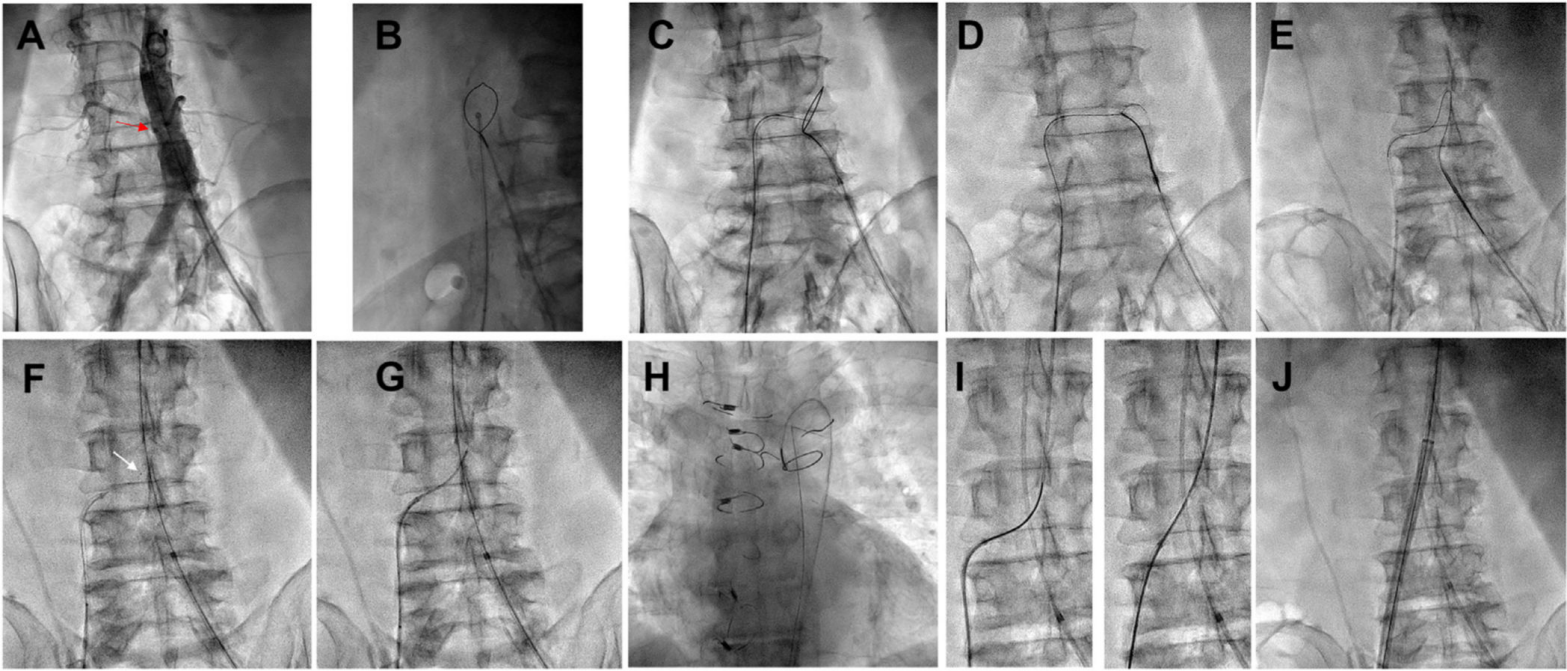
Traversal Sequence Representative traversal sequence. (A) Aortogram in computed tomography–derived projection. No venogram is required. Arrow indicates target. (B) Orthogonal projection confirms that the traversal system aims at the snare. (C) Electrified guidewire exits the inferior vena cava and enters the aorta. Electrification ceases when the wire crosses the snare border. (D) The aortic guidewire is ensnared while feeding forward to avoid outward traction on the aortic catheter. (E) The aortic guide is advanced, leading with a looped guidewire. (F) A 0.014-inch microcatheter (arrow) creates a 0.035-inch caval-aortic rail to deliver a 0.035-inch microcatheter (G). (H) The 0.014-inch guidewire-microcatheters are released from the snare and withdrawn through the 0.035-inch microcatheter. (I) A Lunderquist-style 0.035-inch guidewire advances across the aortic wall (left) and straightens the microcatheter (right). (J) The transcatheter aortic valve replacement introducer sheath advances into the aorta.
